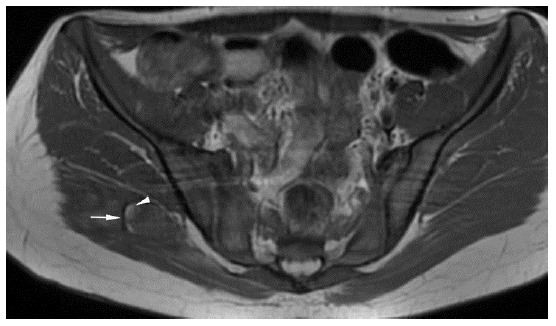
Axial MRI pelvis – fat signal present at periphery of the lesion (arrowhead) adjacent to the rim calcification (arrow) consistent with ossification.
